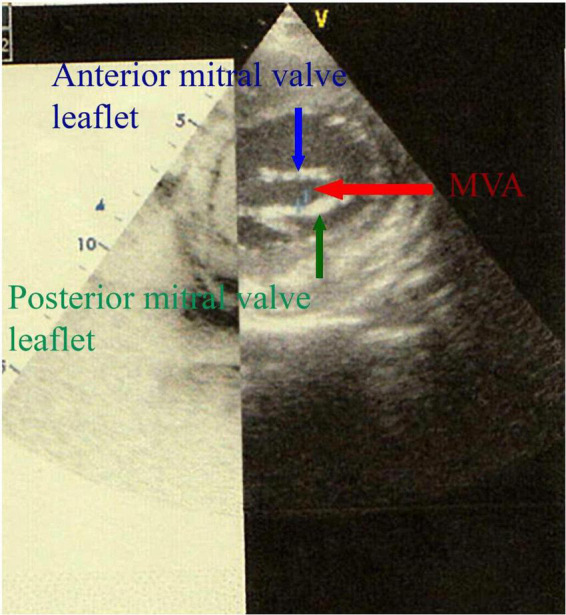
Two-dimensional (2D) transthoracic echocardiography in parasternal short-axis view shows the MVA measurement by planimetry.
